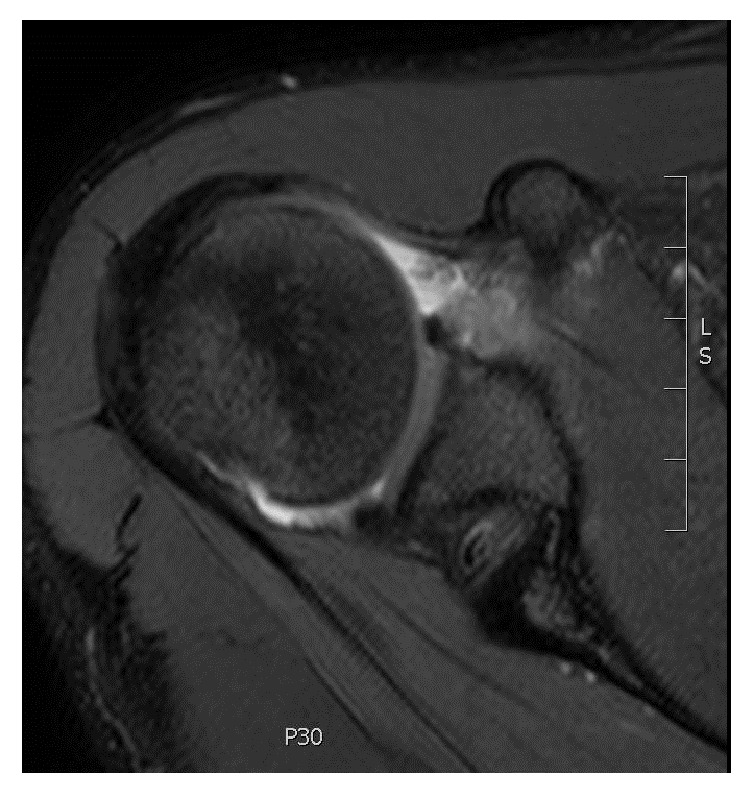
Axial T2 weighted MRI demonstrating absence of the bicipital groove.
